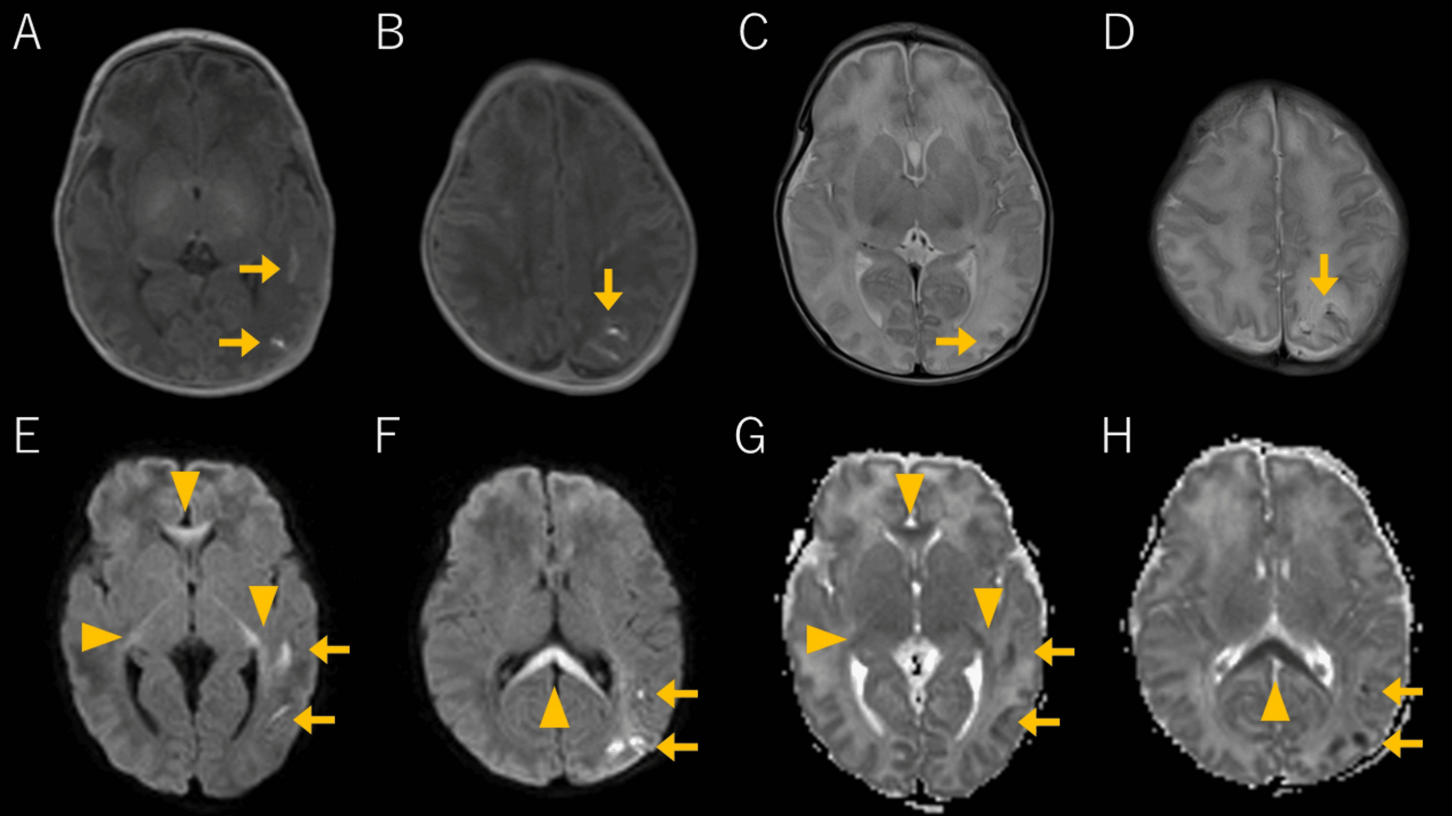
Brain MRI findings on day 6 Subpial hemorrhages are indicated by arrows (→), whereas CLOCCs are marked with arrowheads (▲). Axial T1-weighted images show subpial hemorrhage and cortical-subcortical hematomas with high signal intensity in the left temporoparietal region (A, B). Axial T2-weighted images show high signals in the cerebral cortex and low signal hematomas (C, D). DWI reveals high-signal-intensity lesions in the cortex below the subpial hematoma and reveals the corpus callosum and optic radiation to the posterior limb (E, F). The mean ADC values of the splenium were 663×10^-6^ mm^2^/s (G, H). MRI: magnetic resonance imaging; CLOCCs: cytotoxic lesions of the corpus callosum; DWI: diffusion-weighted imaging; ADC: apparent diffusion coefficient
